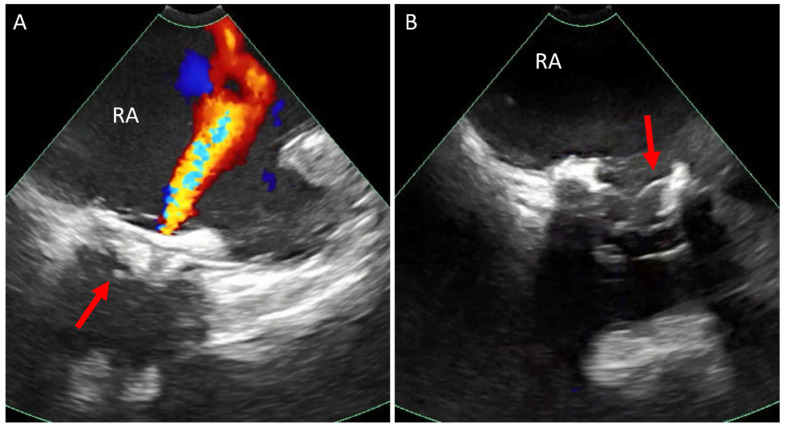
ICE catheter in the right atrium. (A) Color Doppler at the tricuspid valve in a 26-year-old patient with severe prosthetic tricuspid valve stenosis, showing calcified valve (arrow) with at least moderate regurgitation. (B) Color Doppler view in systole post TVIV showing no regurgitation and the new valve leaflets are well seen (arrow). ICE, intracardiac echo; RA, right atrium; TVIV, transcatheter tricuspid valve-in-valve replacement.
